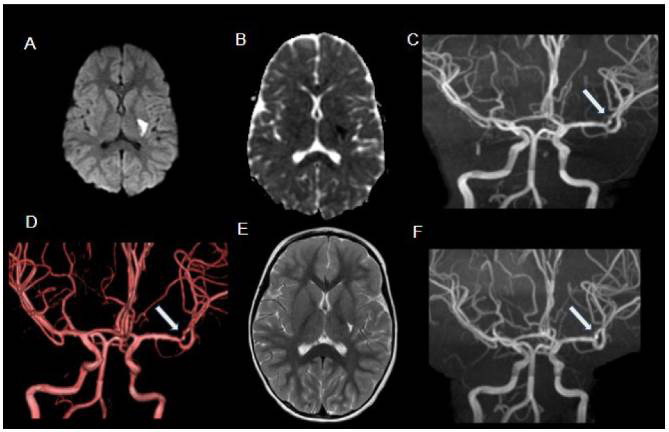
Varicella. Acute stroke in a 3-year-old female patient presenting with lower-limb weakness, weeks after chickenpox. DWI (A) and corresponding ADC map (B) show restricted diffusion in the posterior left putamen and internal capsule. MRA (C and D) depicts irregularities in the M1-M2 segments of the left middle cerebral artery (arrows). Follow-up MRI after 5 months demonstrates residual findings (E, T2WI) and partial resolution of the vascular irregularities (F, MRA, arrow).
